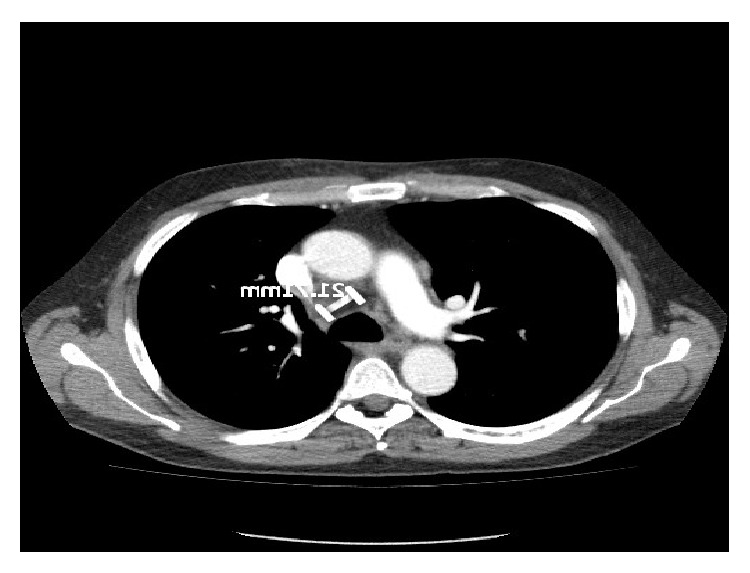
CT scan (pretreatment) showing lymph nodes.
